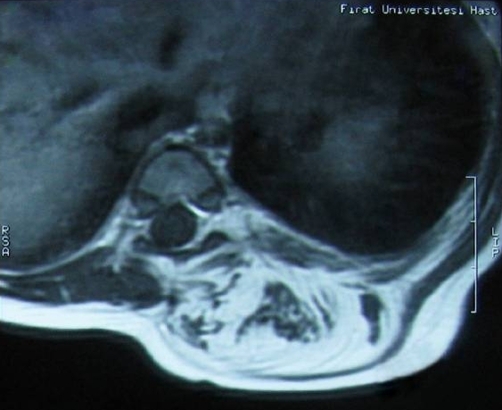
Axial MRI view demonsrating the mass which belonging to lipomyelomeningocele was located to left lateral lower thoracic region. As seen from this axial view, the mass was extending from the paraspinal region to spinal canal and almost deplacing the spinal cord.
